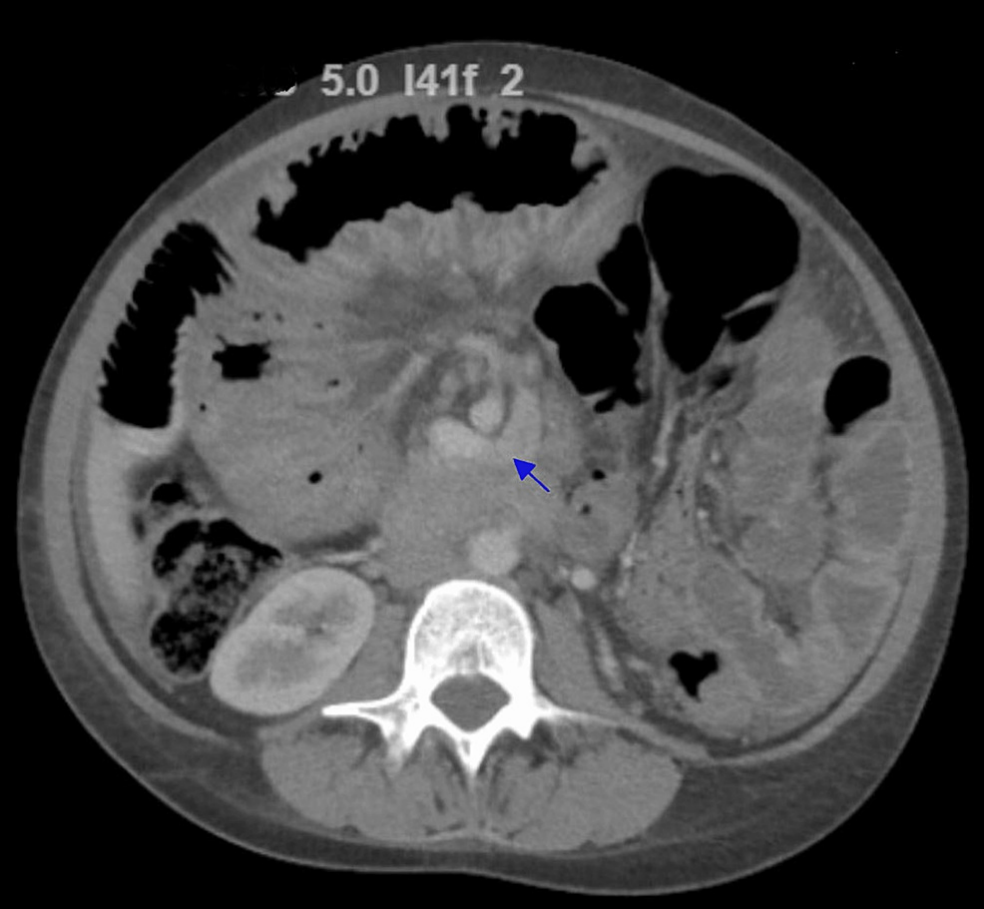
CT of the abdomen with contrast CT of the abdomen showing swirling of the mesentery
